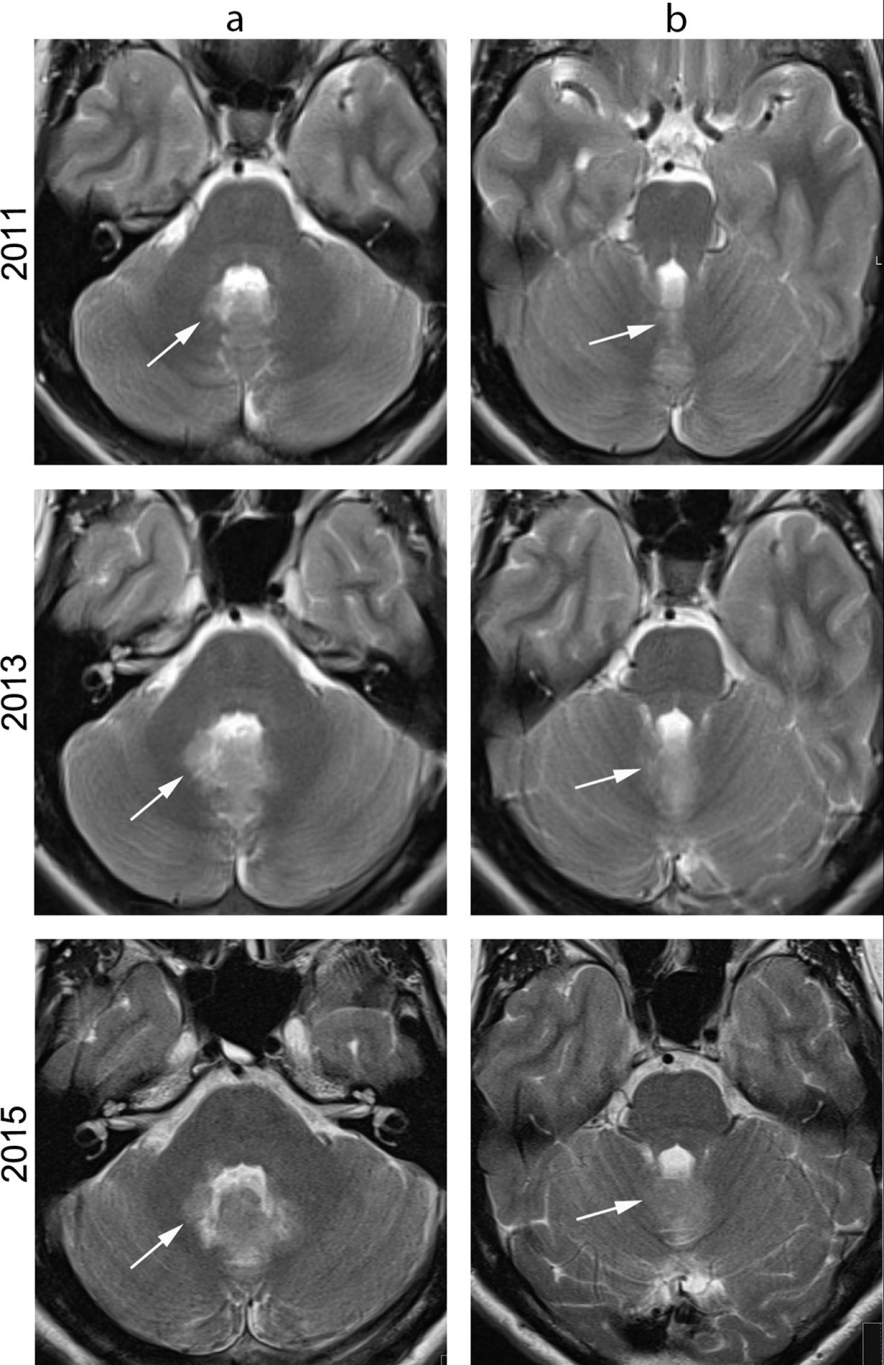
T*_2_ weighted MR images at the level of the pons (a) and at the level of the mesencephalon (b) at first presentation in 2011 (first row), at second presentation in 2013 (second row) and at time of diagnosis in 2015 (third row). In 2015, an abnormal *T*_2_ hyperintense signal is seen on the dorsolateral side of the fourth ventricle (arrows), with little mass effect. Retrospectively, this area could also be seen on previous MRI examinations, albeit less clearly.
